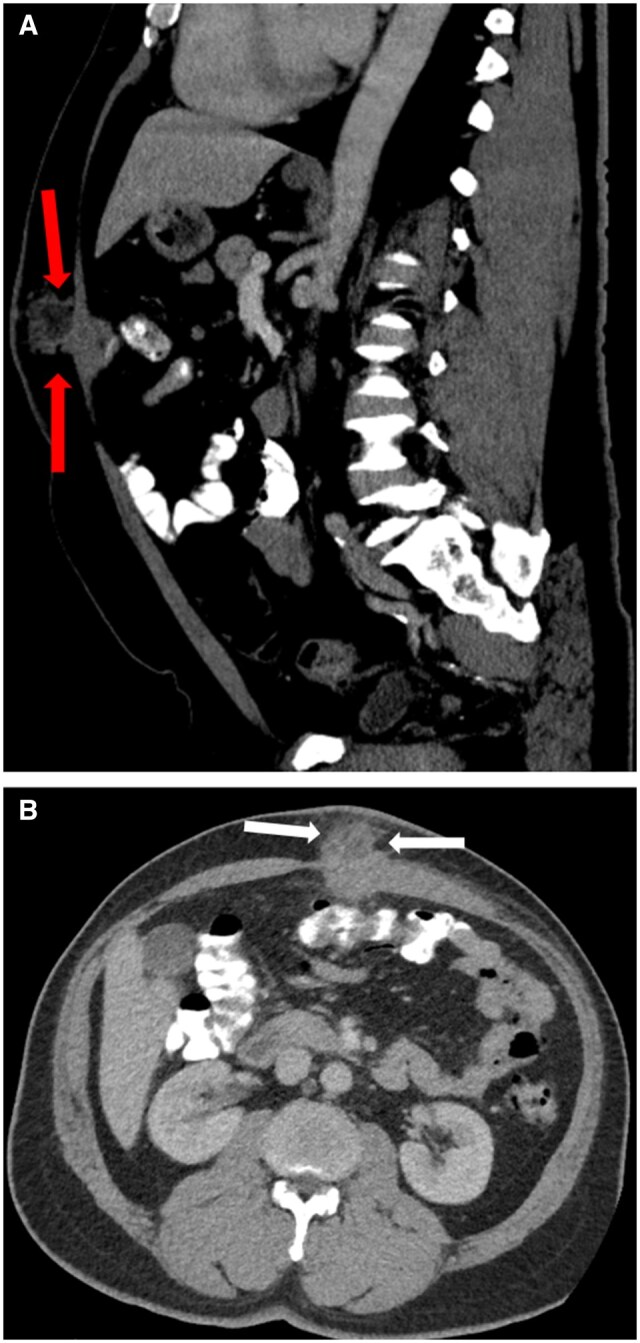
Case 1. Follow-up CT with PV contrast in sagittal (A) and axial (B) views demonstrates post-incision and drainage appearance of the inflammatory mass, which appears less bulky and avidly enhancing compared to Figure 1.
